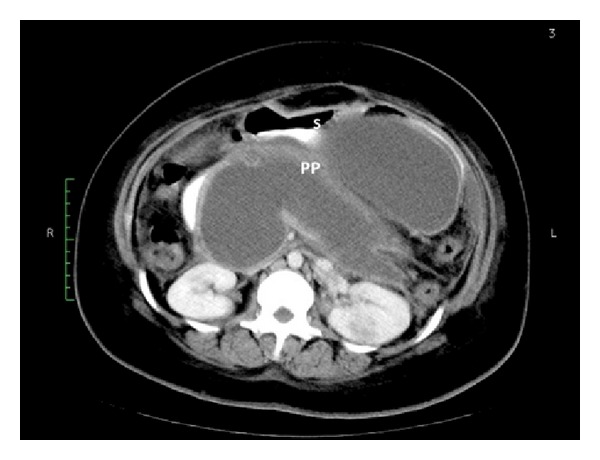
Axial slice of a CT scan of a patient with a large pancreatic pseudocyst (PP) demonstrating its apposition onto the posterior wall of the body of the stomach (S).
